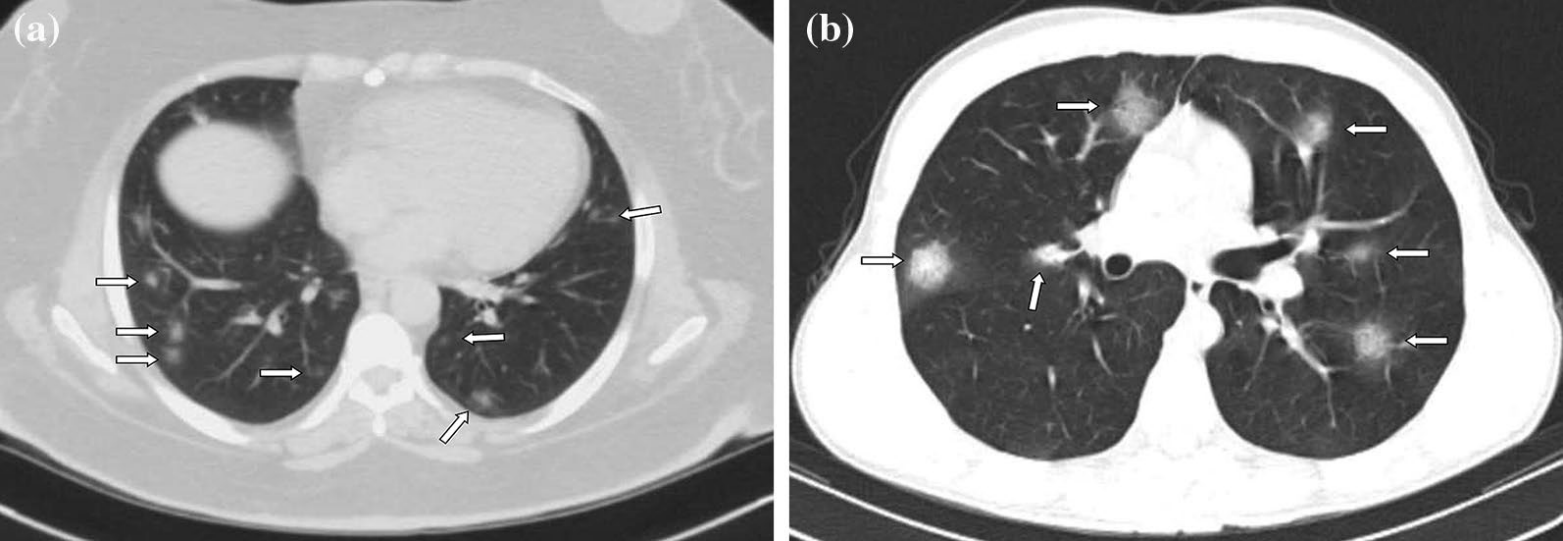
a, b Case examples defined in the probable COVID-19 for the BSTI guidance statement, and in the typical category for the RSNA expert consensus statement. a An axial chest CT image of a 46-year-old female with COVID-19 shows peripheral, bilateral, multifocal, rounded GGOs (arrows). b An axial chest CT image of a 19-year-old male with COVID-19 shows peripheral, bilateral, multifocal, rounded GGOs (arrows)
